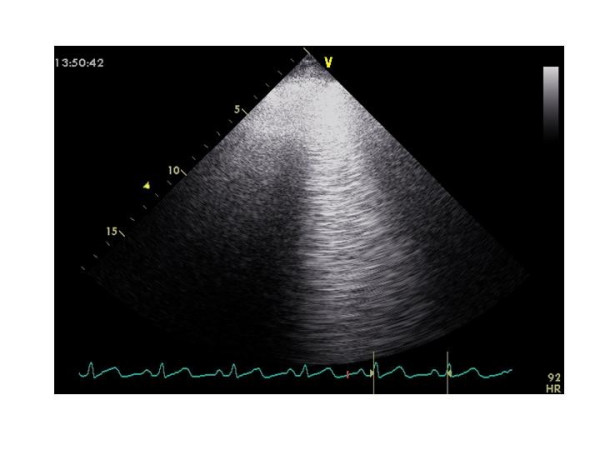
Ultrasound evidence of lung water ("comet tail") of V inter-costal space in a 58-year old male patient with pulmonary microcitoma after CT including carboplatinum, etoposide and radiation therapy.
